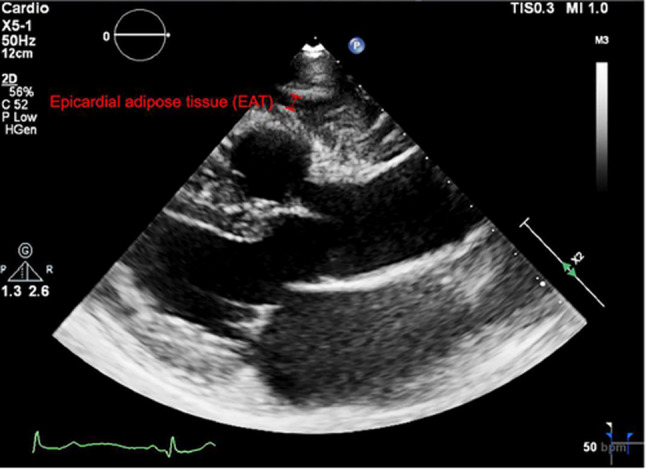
Standardized Assessment of Epicardial Adipose Tissue (EAT) Thickness using Transthoracic Echocardiography
